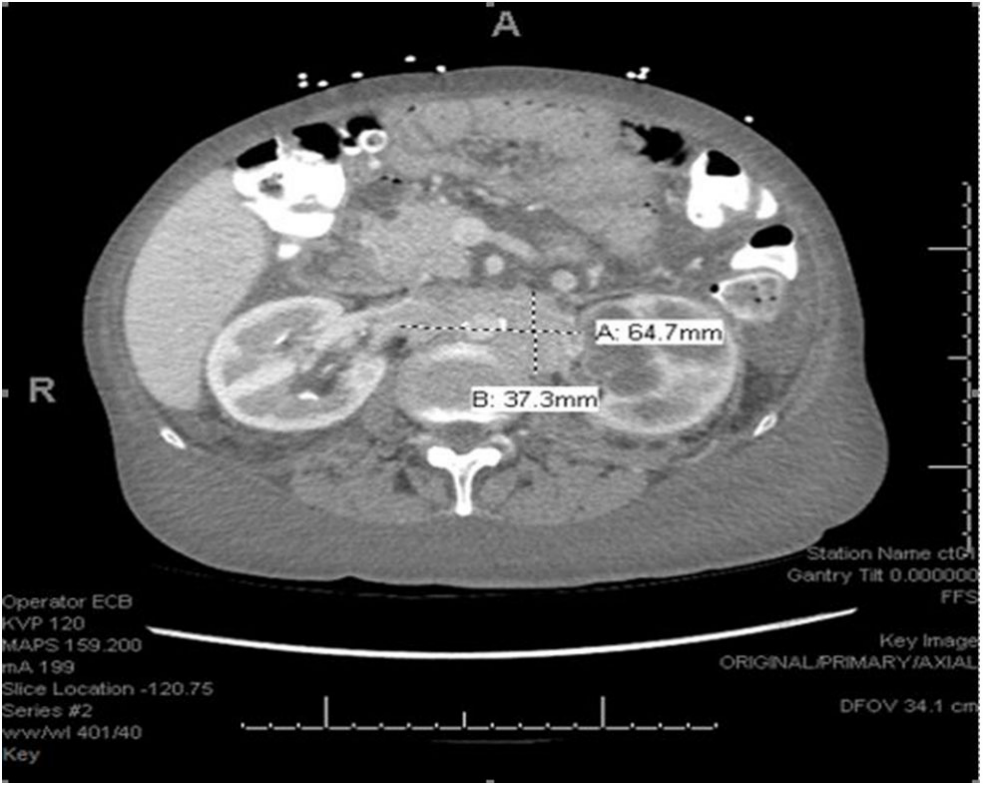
CT scan with extensive retroperitoneal adenopathy.
